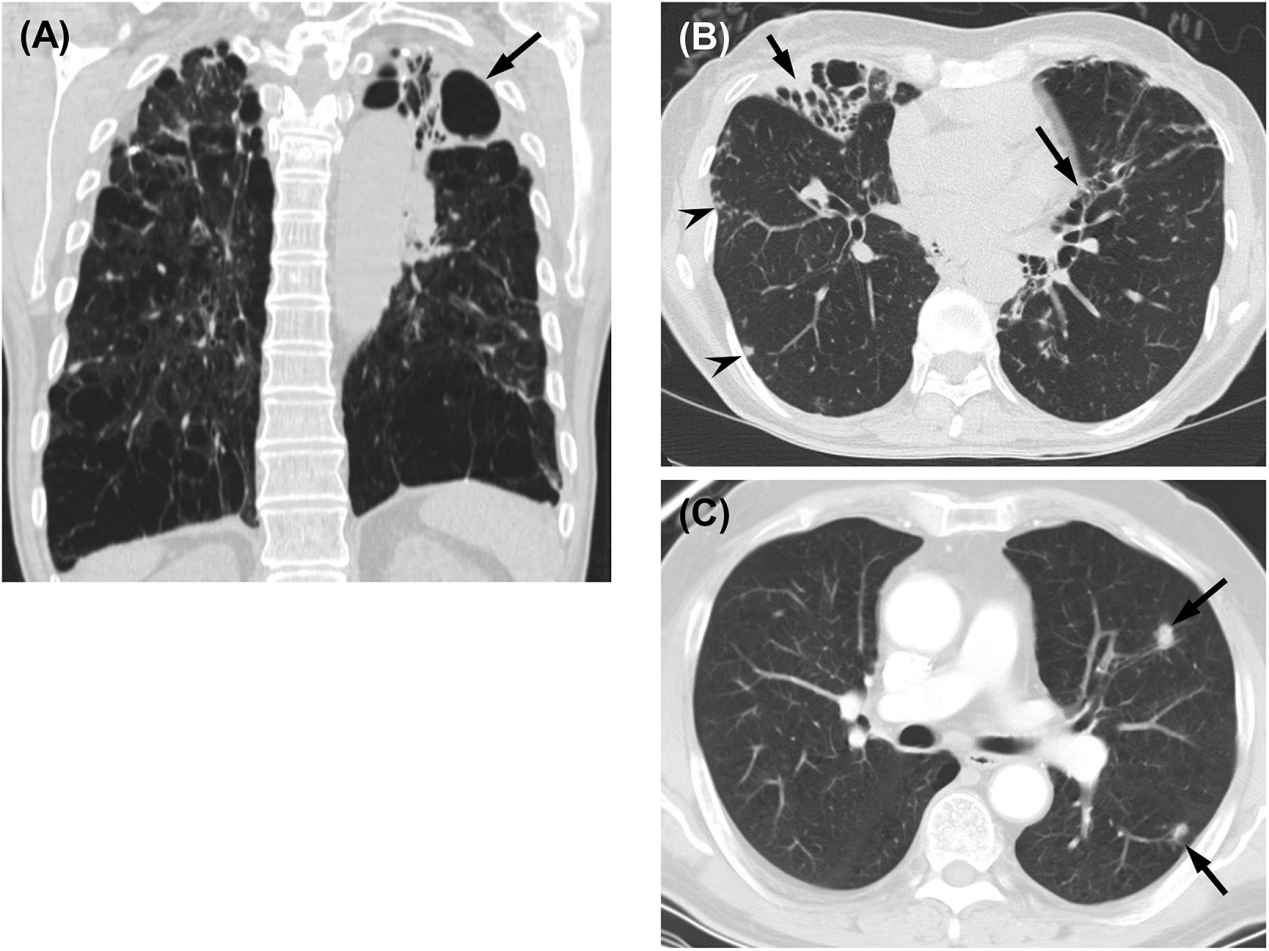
Diverse patterns in images of *M*. *kansasii* lung disease. (A) A 64-year-old man with *M*. *kansasii* lung disease. A coronal thin-section (2.5-mm thickness) CT scan shows a thick-walled cavitary lesion (arrow) with consolidation in the left upper lobe. Severe pulmonary emphysema is also observed. (B) A 66-year-old woman with *M*. *kansasii* lung disease. A transaxial thin-section (2.5-mm thickness) CT scan obtained at the basal trunk level shows bronchiectasis (arrows) and multiple branching centrilobular nodules, the so-called tree-in-bud pattern (arrowheads), in both lungs. (C) A-77-year-old man with *M*. *kansasii* lung disease. A transaxial (5-mm thickness) CT scan obtained at the level of the left main bronchus shows two nodules in the left lung (arrows). Lung biopsy revealed chronic granuloma; *M*. *kansasii* was isolated from the bronchial washing fluid.
